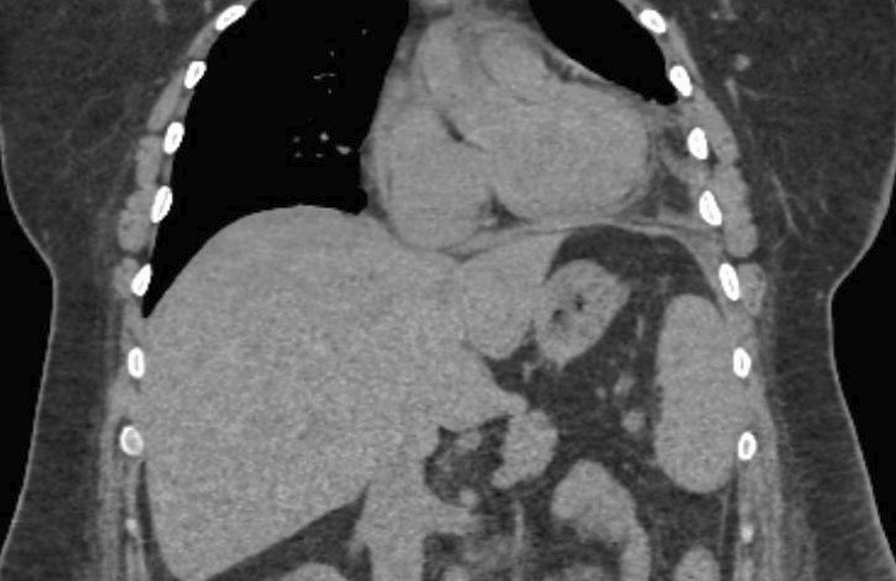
Computed tomography coronal plane images showing a regression of the pericardial effusion and decreased abscess formation
